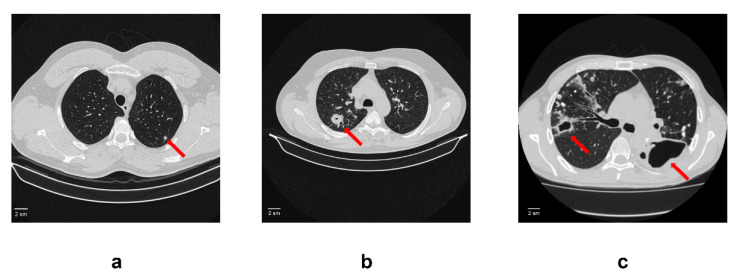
An example of computed tomogram images of active lung TB. (a) Lung tuberculoma without “decay”. In S2 of the left lung, there is a rounded formation with clear contours 5 mm in diameter (red arrow); (b) Lung tuberculoma with “decay”. In S2 of the right lung, there is a rounded formation 2 cm in diameter with a small decay cavity in the center (red arrow). In the surrounding tissue, single foci and fibrous changes; (c) FCT. In the right lung in S2, there is a decay cavity with irregular contours and infiltration around the walls (red arrow). The decay cavity is drained S2. In the rest of the upper lobe, calcifications and foci with infiltration and decay. On the left, there is a large decay cavity in S6 with uneven contours (red arrow). Limited empyema of the pleura on the left in the S6 projection cannot be ruled out.
